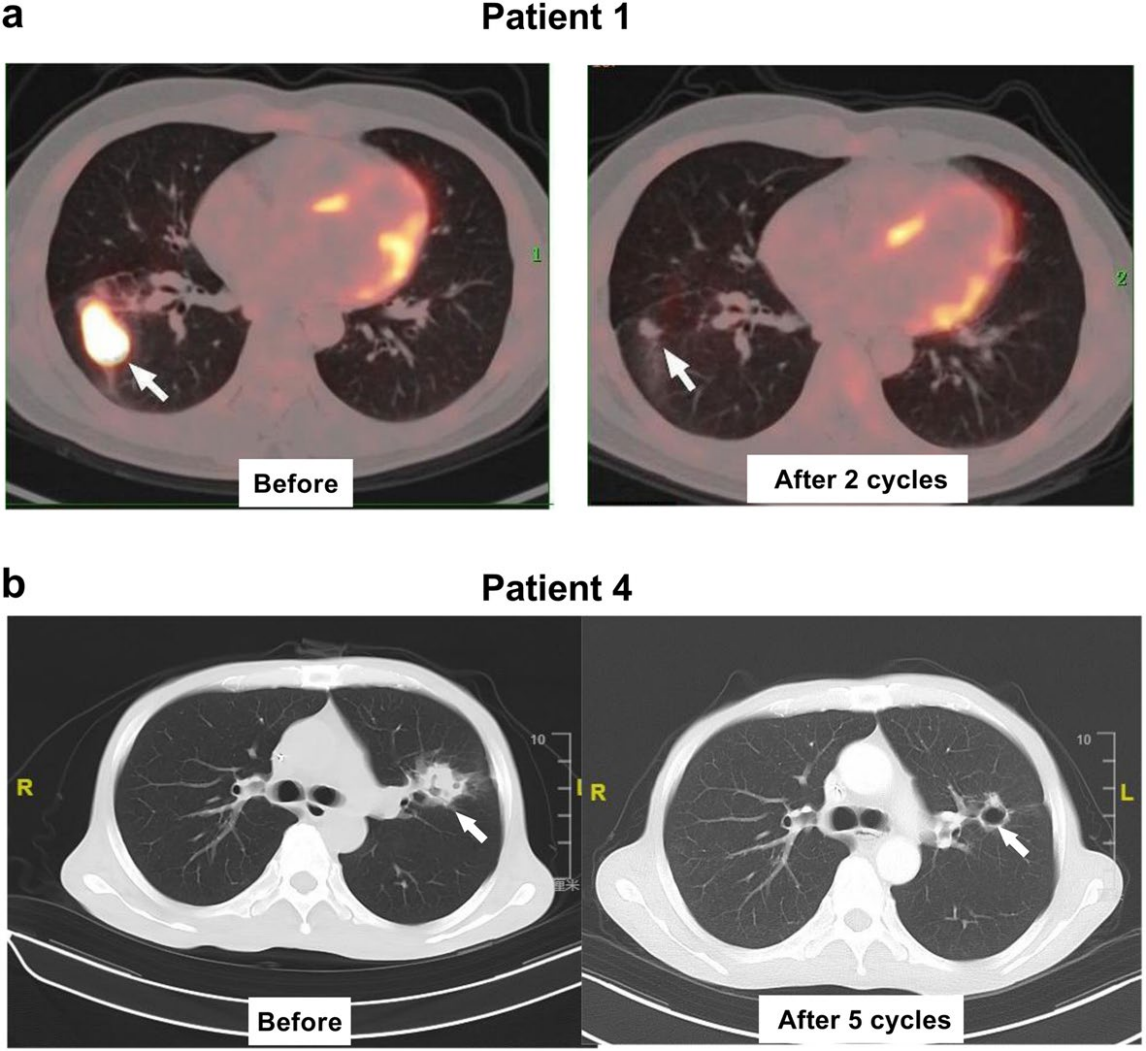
Pre- and post- programmed death-ligand 1 (PD-1) inhibitors plus chemotherapy treatment response on positron emission tomography (PET)/computed tomography (CT) or CT scans (a) Patient 1: Significant reduction in right lung lesion (white arrow) and complete response on PET/CT after two cycles (b) Patient 4: Most lesions (white arrows) undetectable after five cycles
